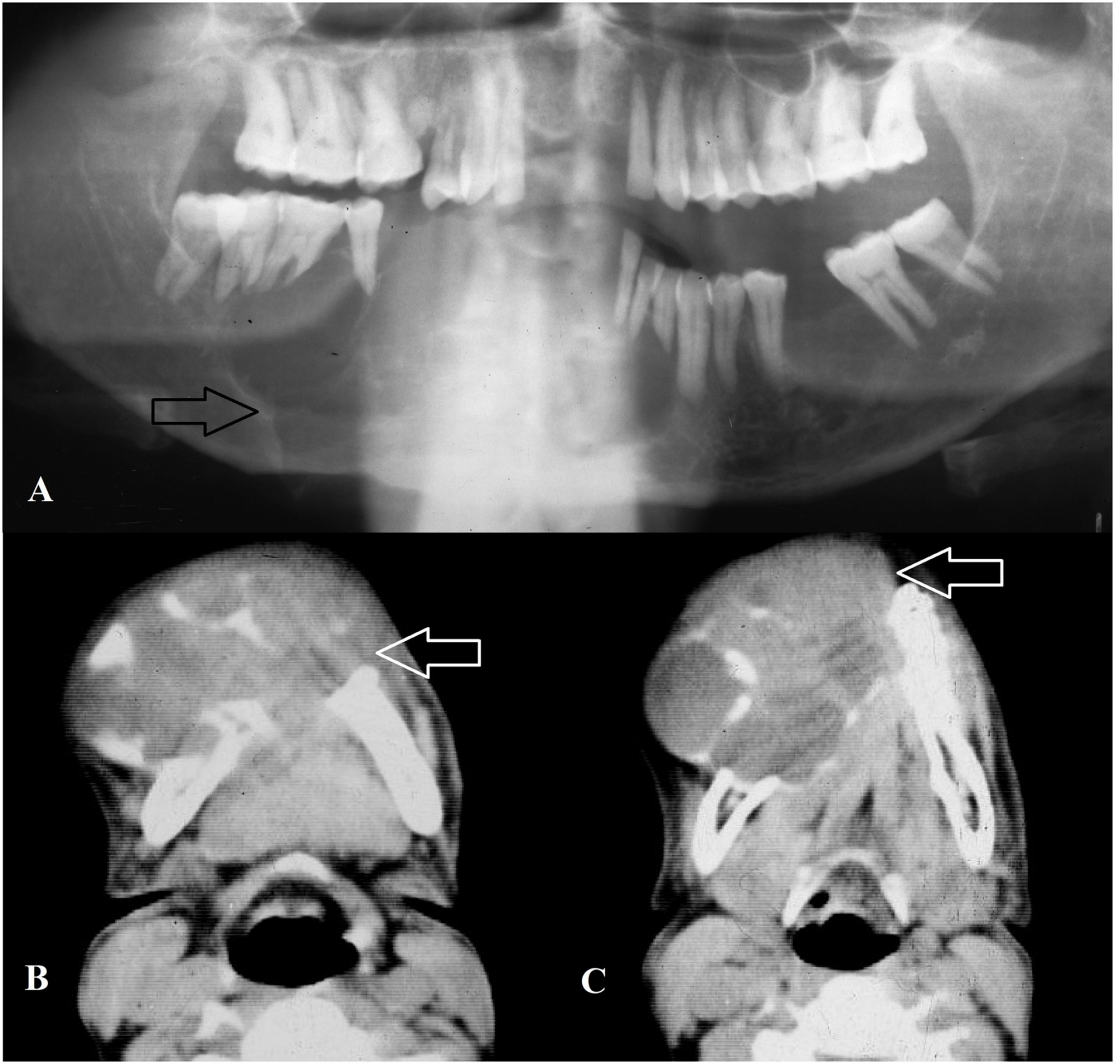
A: Panoramic radiograph showing destructive, ill-defined squamous cell carcinoma arising from the epithelial lining of residual cyst in the mandible. B and C: Axial computed tomography images of same patient showing invasive, expansive and multilocular lesion in the mandible.
